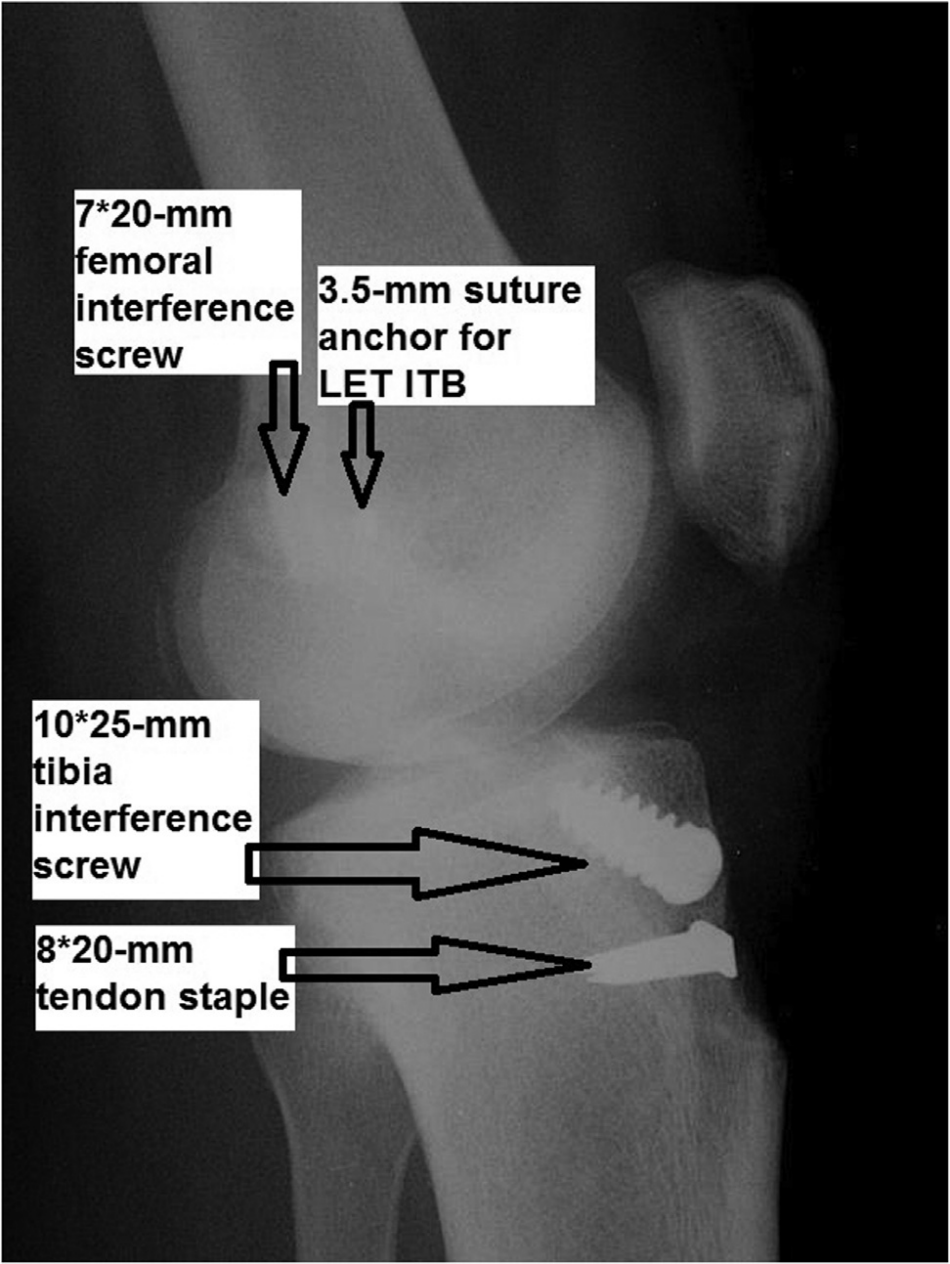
Postoperative lateral radiograph of the left knee showing hardware locations and confirming patella integrity.
